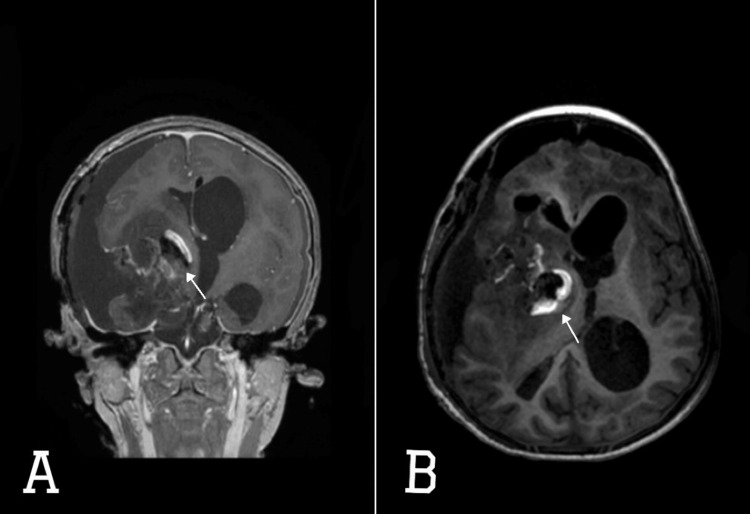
MRI brain with contrast (A) axial and (B) coronal views showing right frontoparietal craniotomy with tumor resection showing ending postoperative change with blood degradation product and enhancing rim (white filled arrows), edema, and air seen within surgical bed, right cerebral convexity fluid collection of maximal thickness of 1.3 cm, pneumocephalus in the right lateral frontal convexity and frontal horns, small layering of intraventricular hemorrhage seen at the right lateral occipital horns. No significant changes of the midline shift to the left side measures 1.1 cm. Slight improvement of the infratentorial uncal herniation. Improvement of the right lateral ventricle dilatation and mild decreased dilatation of the left lateral ventricle.
